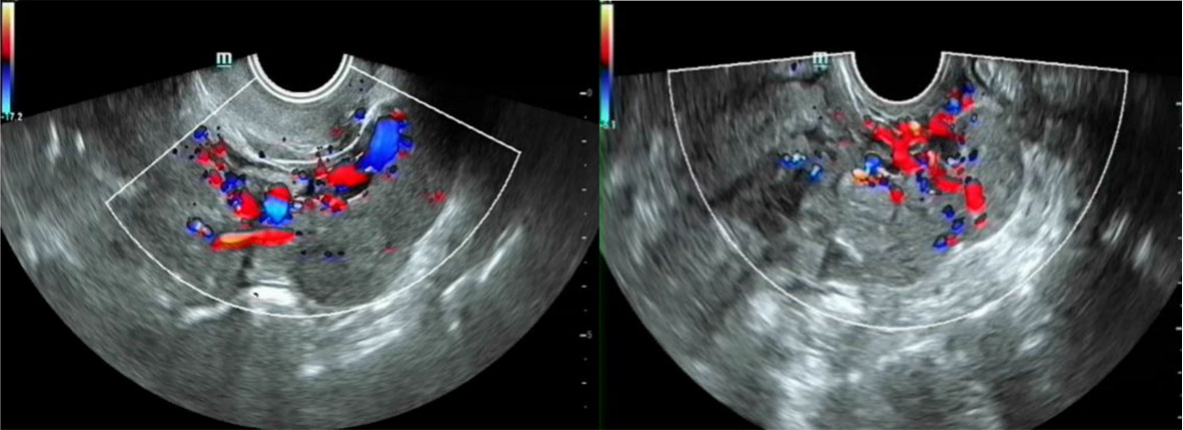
Contrast-enhanced ultrasonography imagine of case 2.
